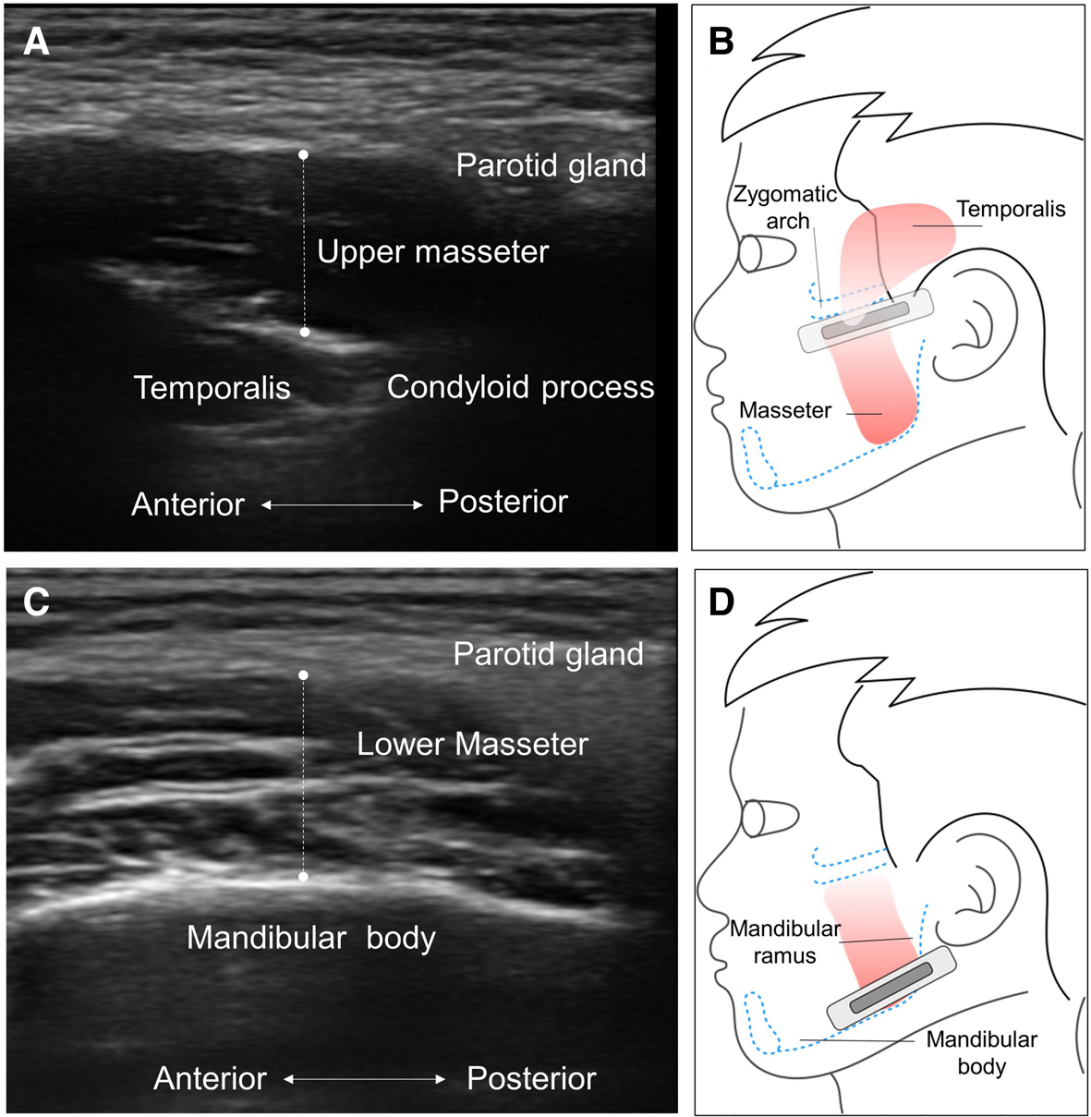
Ultrasound imaging (A) and transducer placement (B) for scanning the upper masseter muscle. Ultrasound imaging (C) and transducer placement (D) for scanning the lower masseter muscle. Note that the length of the dashed lines indicates the thickness of the muscle.
